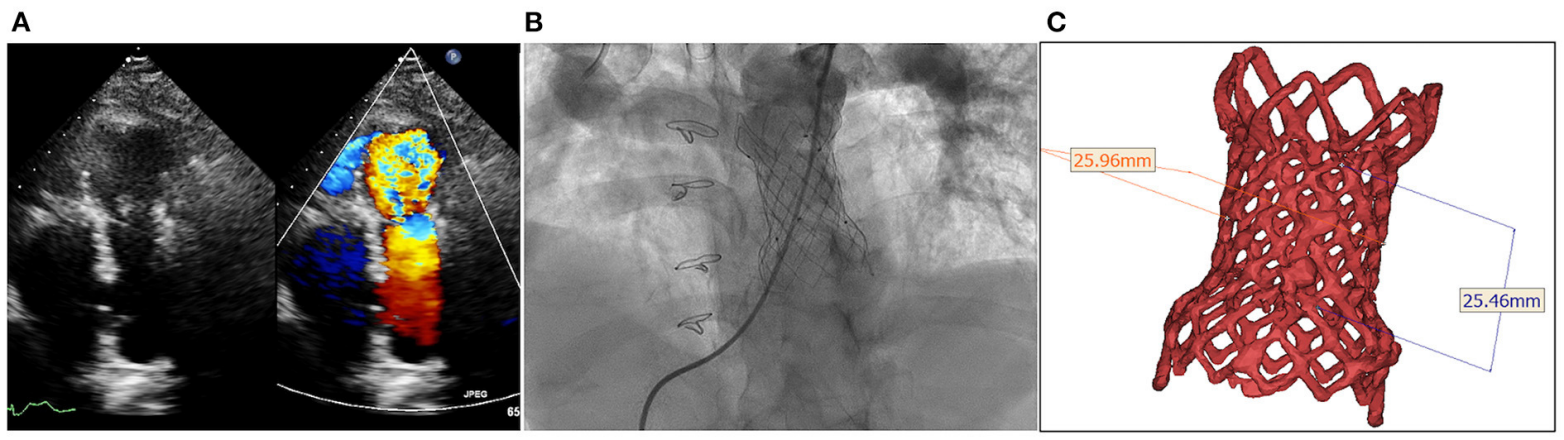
Degenerated bioprosthesis with complete frame. (A) Transthoracic echocardiography shows severe pulmonary regurgitation; (B) Angiography reveals severe pulmonary insufficiency; (C) The annular size calculated on computed tomography.
